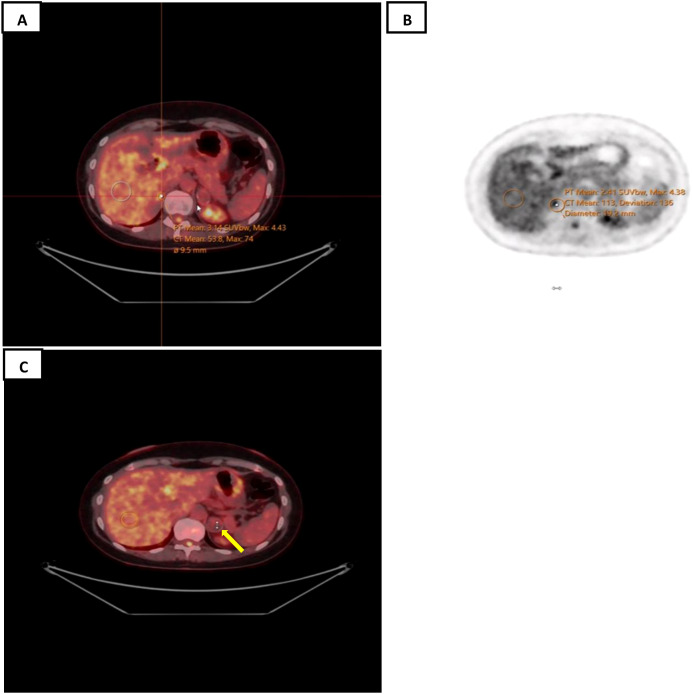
(A, B) Whole body ^18^F-FDG PET/CT scan showed suspicious mild focal activity within the non-enlarged right adrenal gland with tiny calcification. (C) Non-FDG-avid peripherally calcified lesion in the left adrenal gland measuring up to 2.3 cm (*yellow arrow*).
